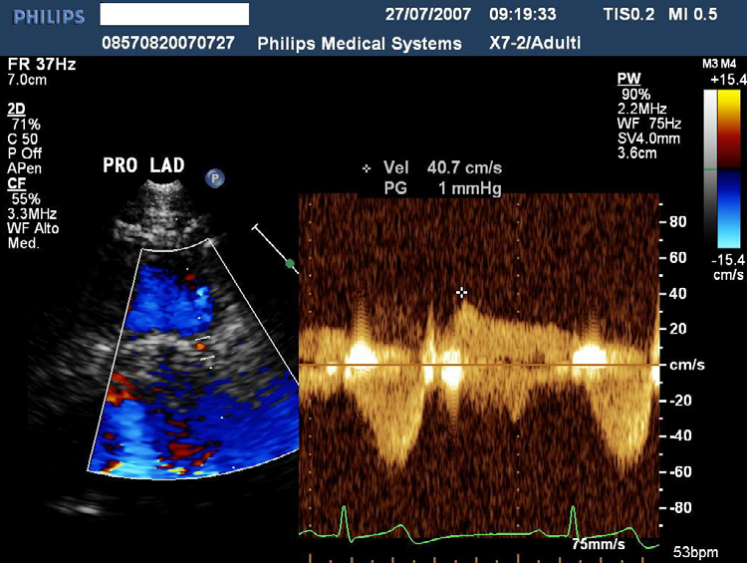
shows the pulse Doppler envelope of proximal tract of the left descending coronary artery.
